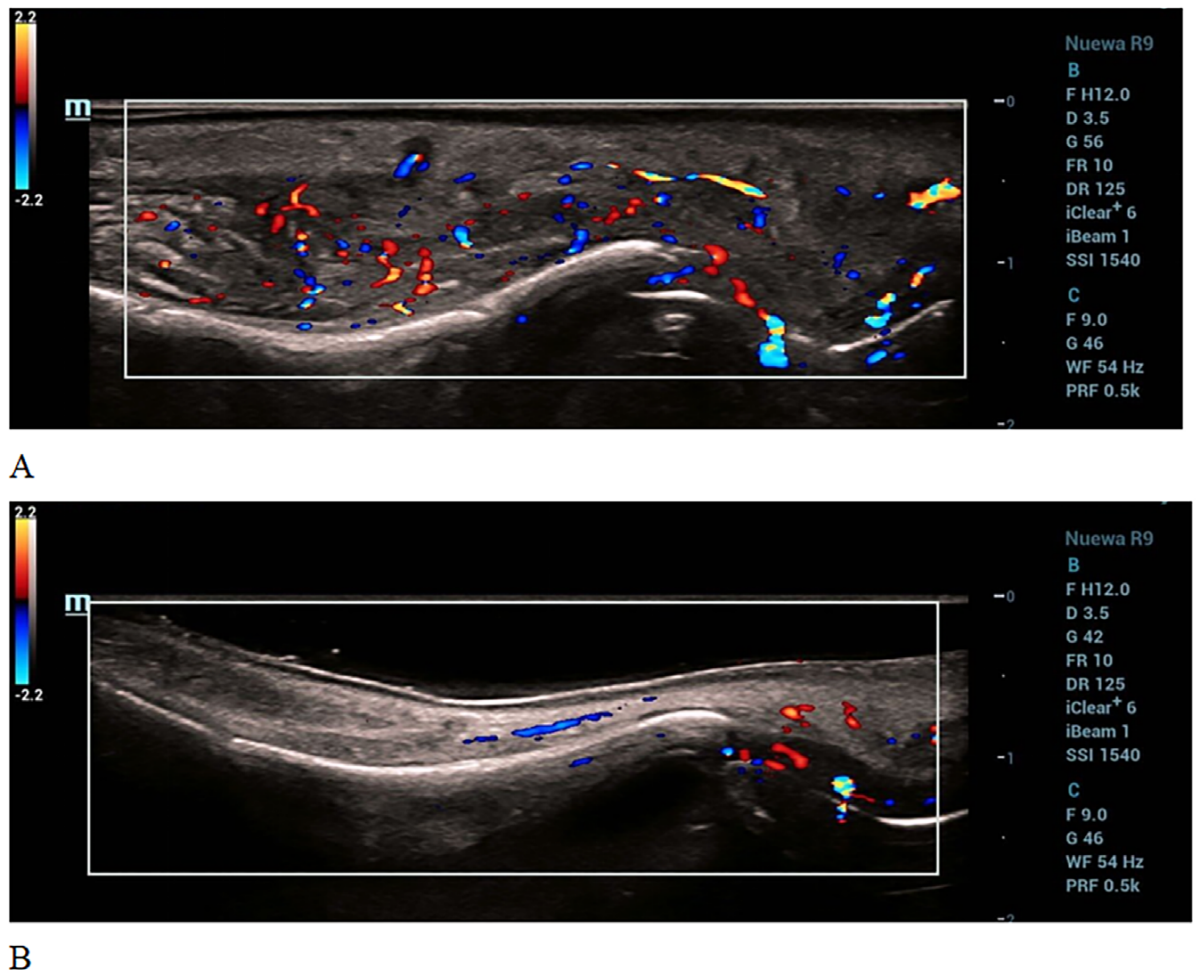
Facial color ultrasound images of Case 2. (A) Before treatment, thickening and chaotic echogenicity are observed in the subcutaneous soft tissue of the nose, mainly in the nasal root area, with a thickness of approximately 1.2 cm. The echogenicity of the deep soft tissue in this area is reduced, with the thickest part measuring approximately 0.9 cm. The surrounding soft tissue shows enhanced echogenicity. Irregular low‐echogenicity areas with unclear boundaries and fine punctate echogenicity inside are visible. Compression appears to cause floating. Abundant blood flow signals are detected in the soft tissue of the nose. (B) After 10 days of treatment, the subcutaneous structures at the root of the nose remain clear. However, the echogenicity of the subcutaneous soft tissue is poor. No obvious abnormal blood flow signals are observed.
